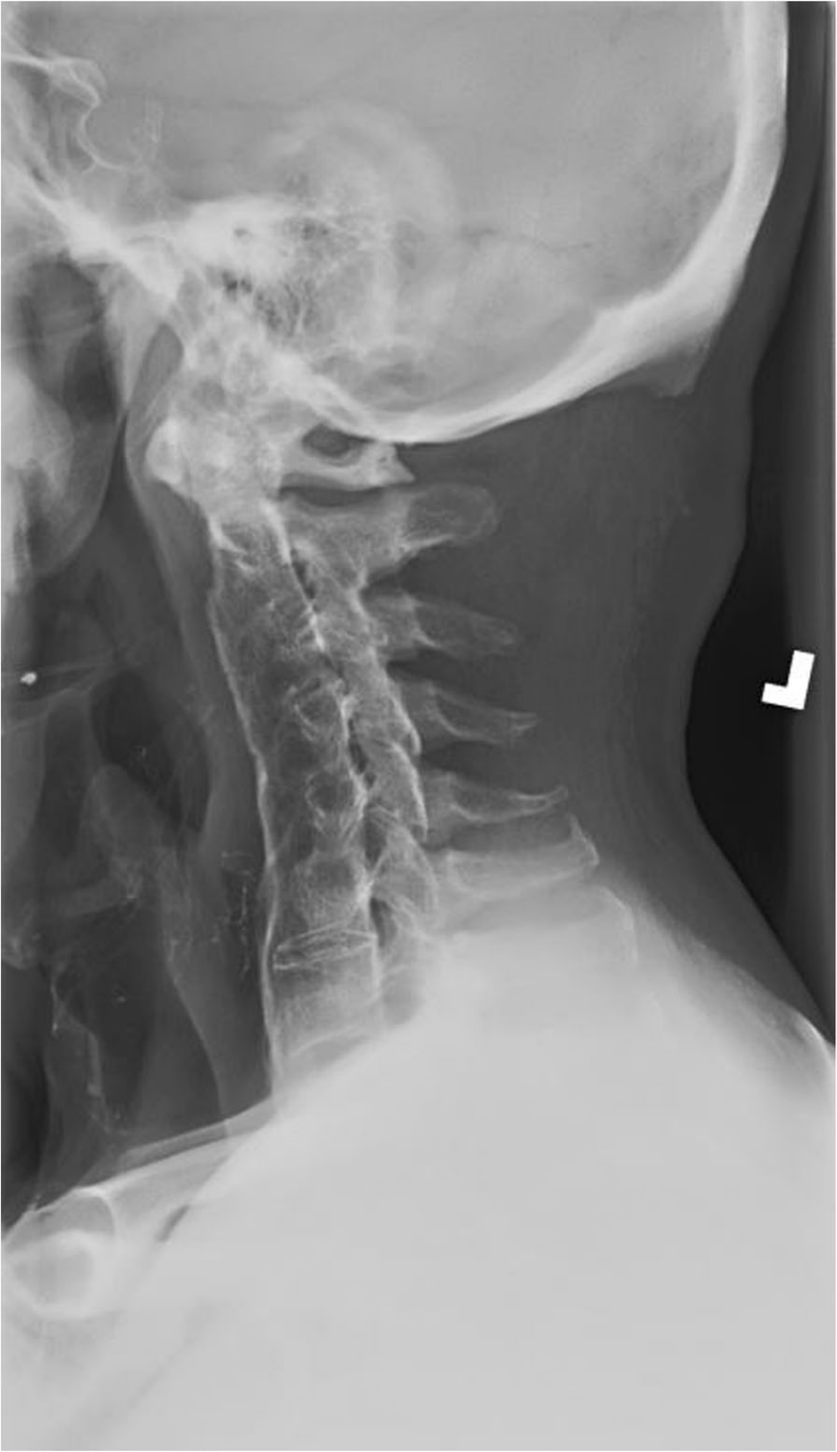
Lateral Cervical Plain Films
